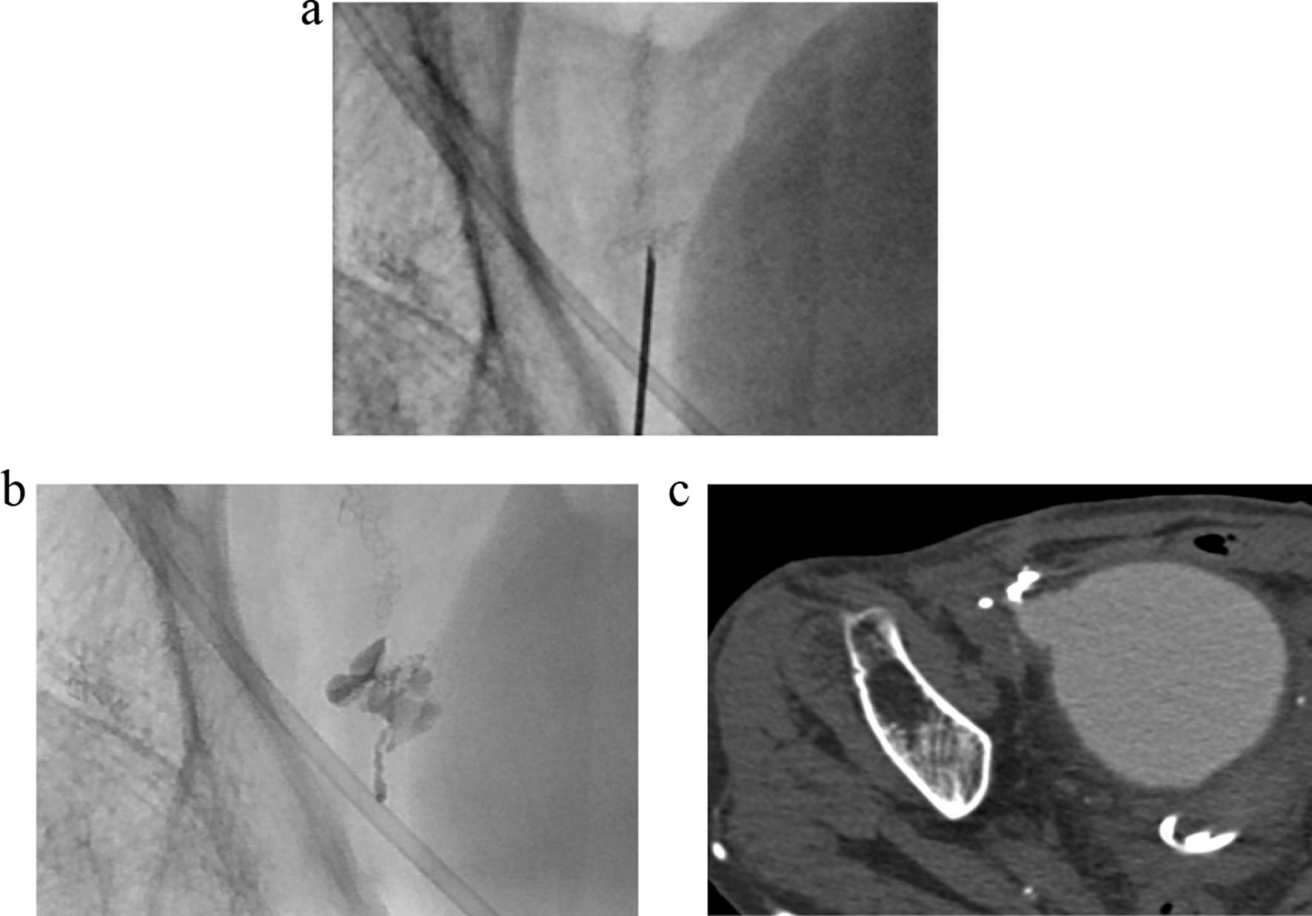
Percutaneous direct injection of *N*-butyl-2-cyanoacrylate (NBCA). a A 22-G needle tip is percutaneously advanced under CT-guided fluoroscopy to the site of the leak using the intersection of the surgical staples (arrow) as a marker. b Immediately after the injection of 0.1 ml of 50% NBCA, a fluoroscopic image shows excellent retention of the NBCA cast at the leak site (arrow). c A CT image obtained immediately after the injection of 0.1 ml of 50% NBCA shows excellent retention of the NBCA cast at the leak site (arrow)
